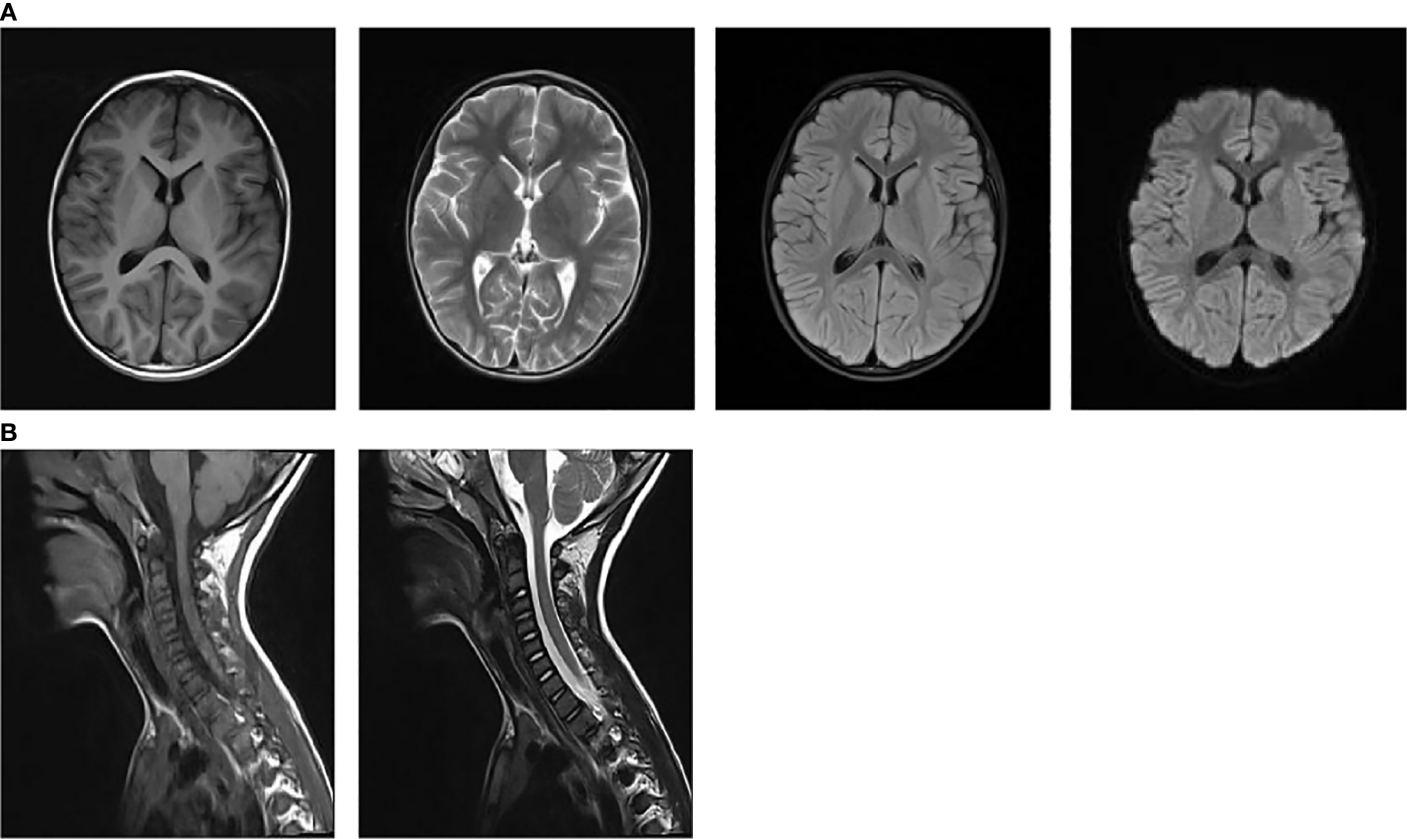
The head (A) and the cervical spinal cord (B) MRI examination was negative.
